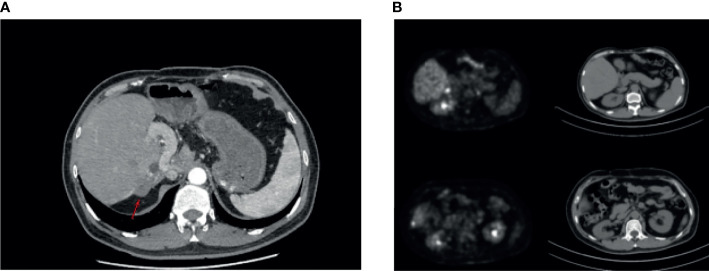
(A) October 22, 2019: magnetic resonance cholangiopancreatography showed the density of the soft tissue under the capsule of the right lobe of the liver. The possibility of metastasis was considered. (B) November 4, 2020: discovery of new lesions; tumor metastasis was considered (PET/CT showed nodular thickening of the capsule of the right posterior lobe of the liver, soft tissue density nodules in the fat space above the duodenum on the right side of pancreatic head in the abdominal cavity, and abnormal increase of glucose metabolism). Compared with the previous film, it was a new lesion. A high possibility of tumor metastasis was considered.
